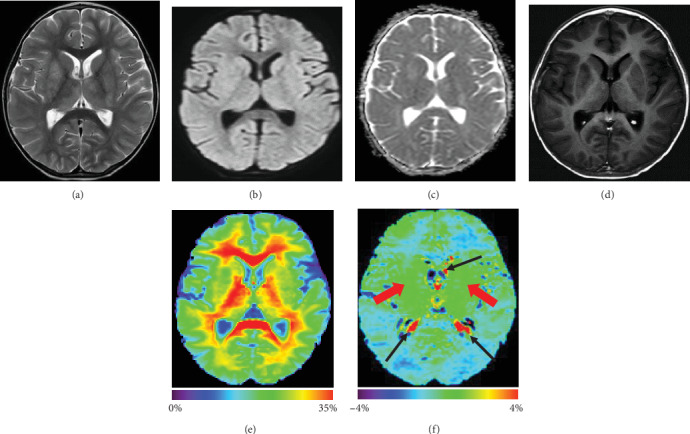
APTw, MTR, and conventional MR images for a 3-year-old boy with viral encephalitis. T2w image (a) demonstrates symmetric increased signal intensity involving bilateral basal ganglia and thalami. DWI (b) and ADC map (c) show no restricted diffusion. The lesions show no enhancement on Gd-T1w (d) and isointensity on MTR (e) and APTw (f) images (red arrows). Note the presence of artifacts in the ventricles (black thin arrows).
